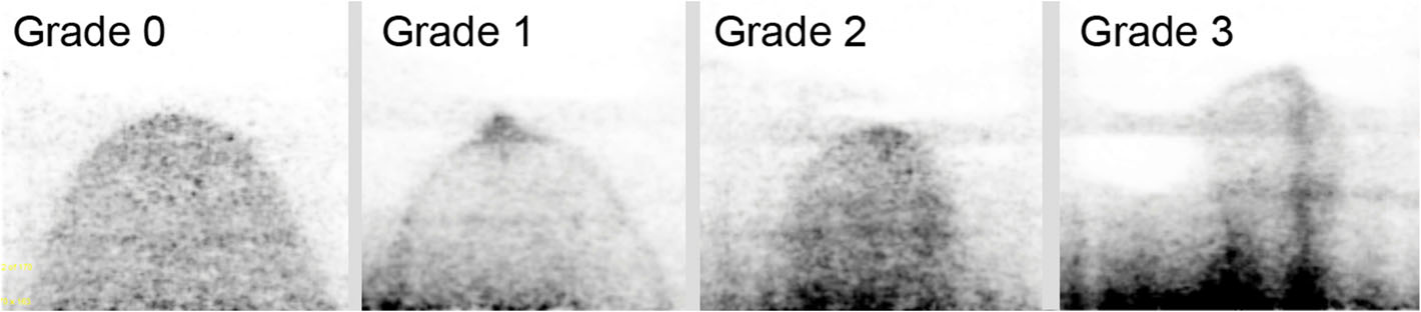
Transaxial images from 4 patient studies demonstrating increasing cross-talk from F-18 FDG in the heart into the breast images, ranging from grade 0 (no cross-talk) to grade 3 (severe increase in background activity impacting overall interpretation of the breast images)
